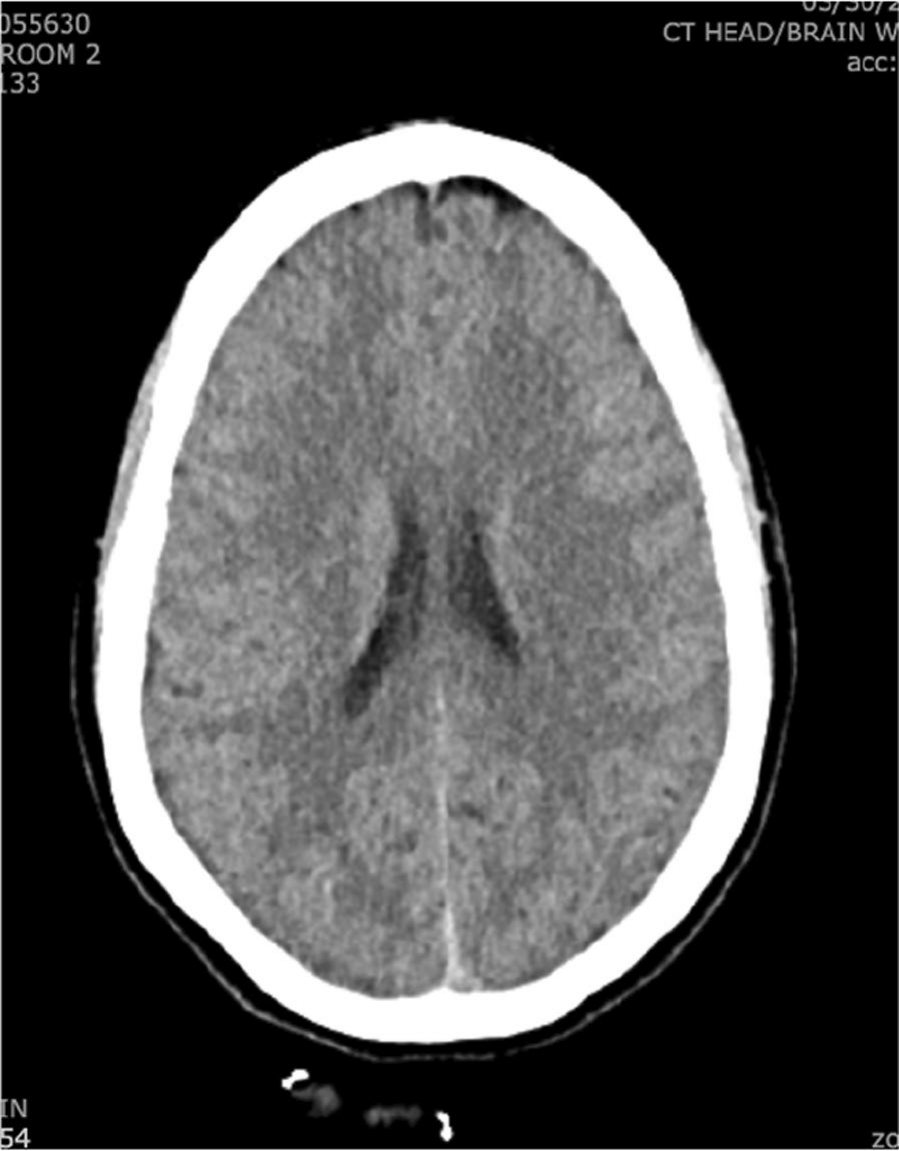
CT head without contrast showed no evidence of intracranial process including masses, bleeding, or elevated intracranial pressure
